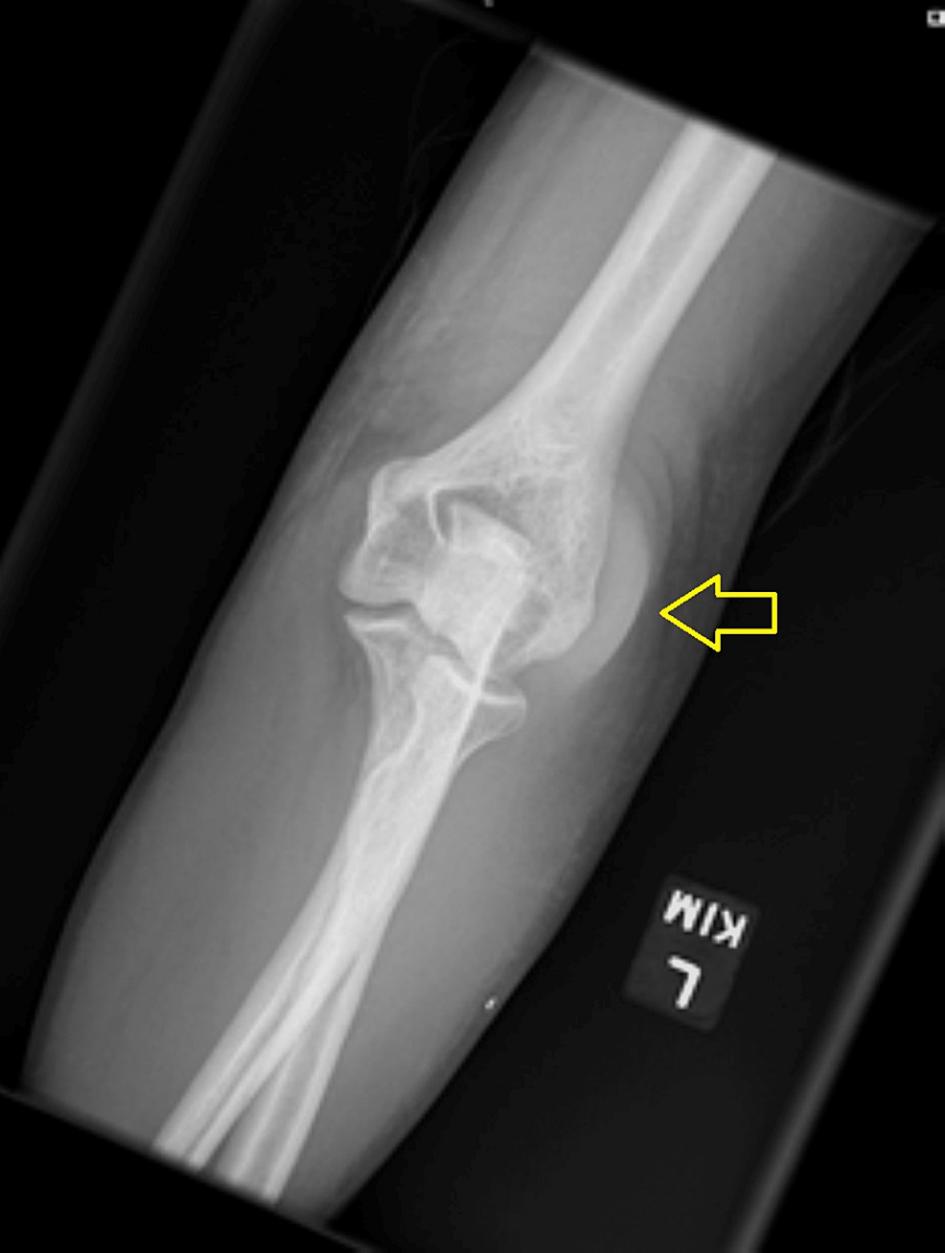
X-ray of left elbow suspicious for olecranon bursitis The arrow indicates the location of olecranon bursitis.
